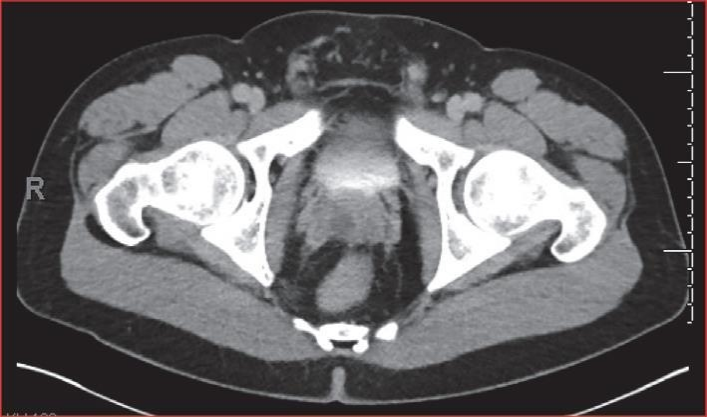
CT of pelvis reveals 1.7 cm fluid attenuation lesion within the right aspect of the prostate gland.
